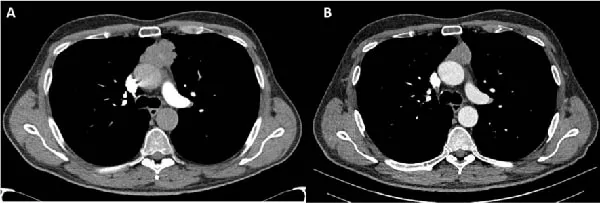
CT staging images before (A) and after (B) neoadjuvant therapy.
